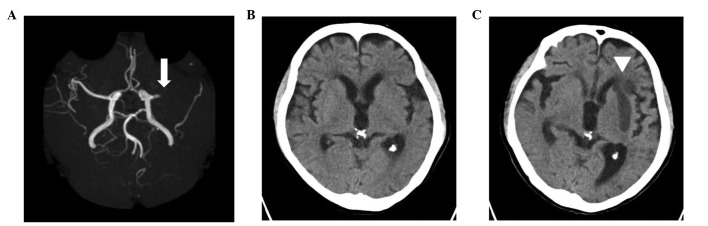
(A) Brain magnetic resonance angiography showing an occlusion of the left middle cerebral artery (MCA) (arrow) on the day of stroke. (B) Brain computed tomography (CT) revealed no new abnormalities on the day of the stroke. (C) CT scan 6 days after the stroke revealed the presence of an infarction in the supply area of the MCA (arrowhead).
